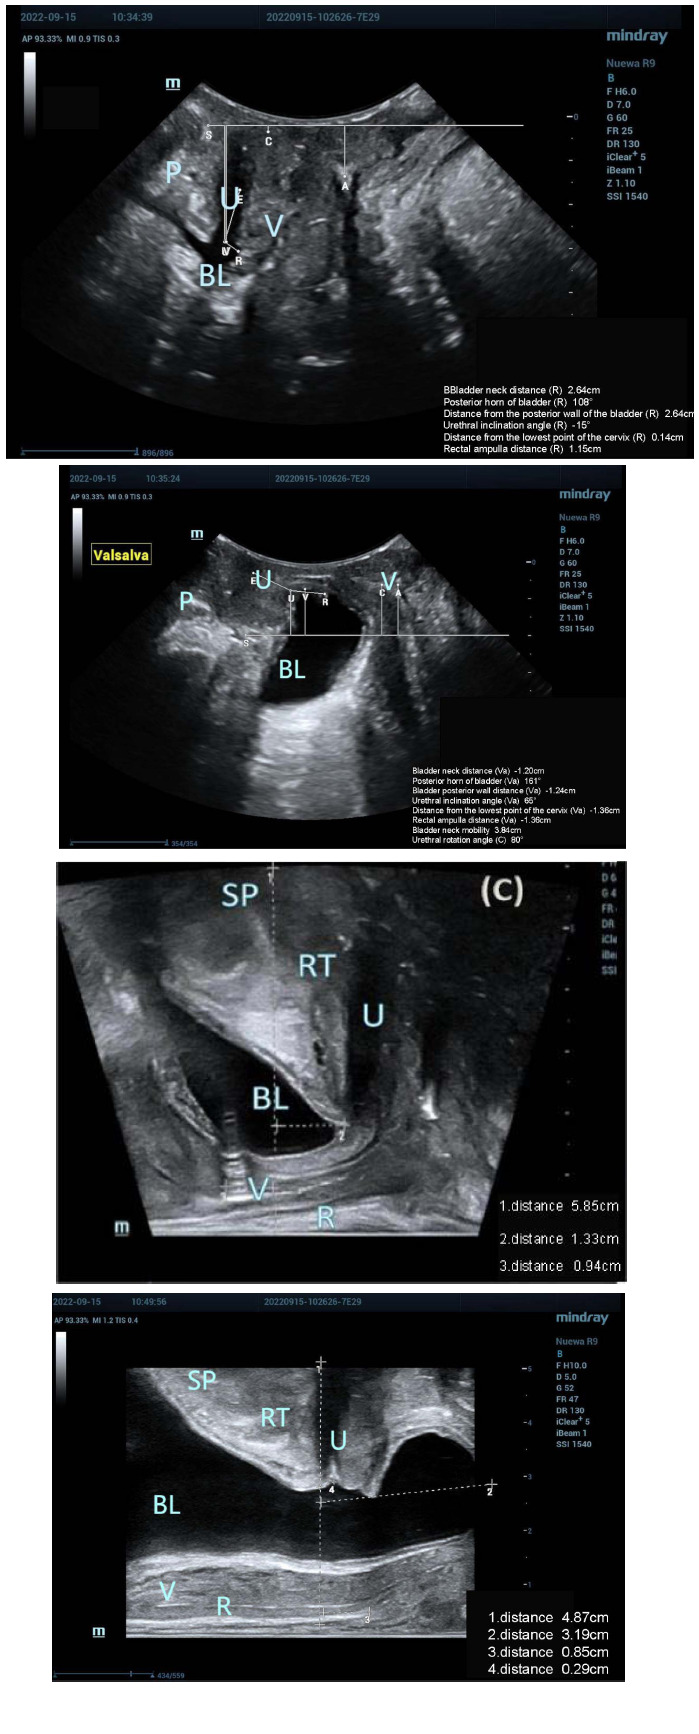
Ultrasound images of the study participants with SUI using the two ultrasound methods.
